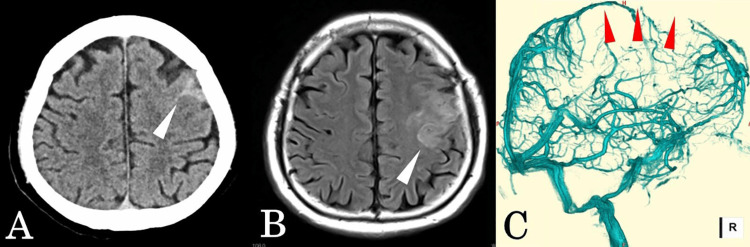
Imaging findings on admission (A) Head CT showing subarachnoid hemorrhage in the left frontal region (white arrowhead). (B) Head MRI showing high signal intensity in the left frontal lobe on FLAIR imaging (white arrowhead). (C) Cerebral angiography in the venous phase showing non-visualization of part of the superior sagittal sinus (red arrowhead) with delayed cortical venous drainage. FLAIR, fluid attenuated inversion recovery
